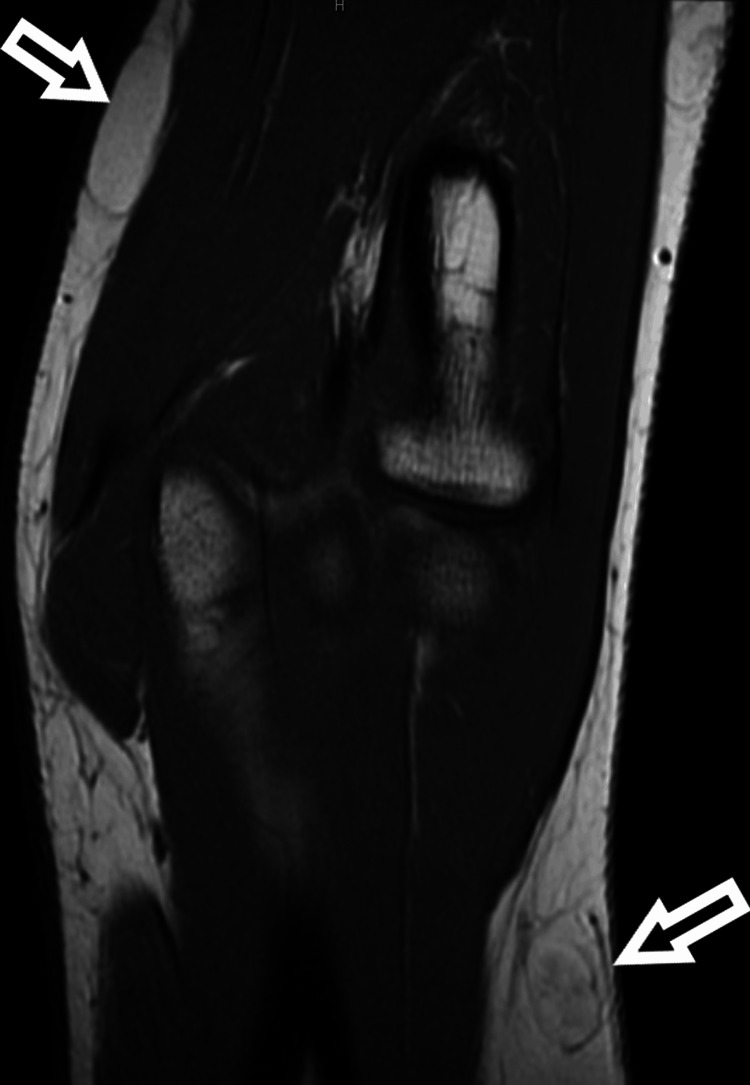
Sagittal T1-weighted MRI of the right elbow demonstrating multiple soft tissue masses in the brachial and antebrachial regions with varying degrees of non-adipose tissue.
